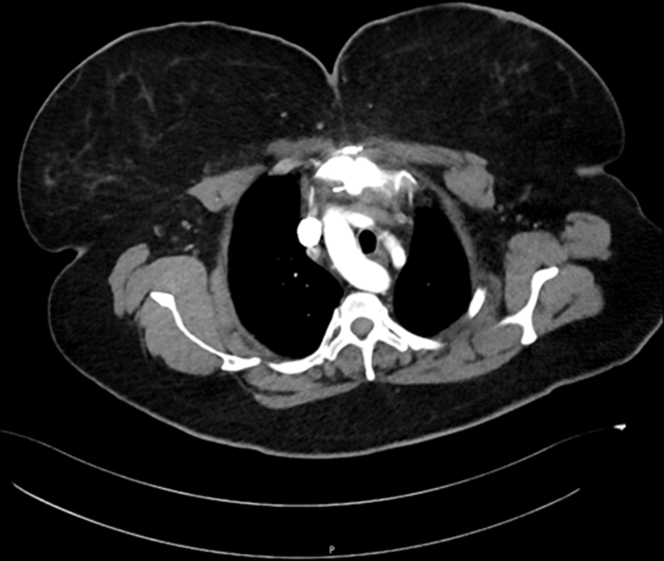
Computed Tomography Scan 6 Months Postoperative Noting Ligation of the Distal Aortic Arch Without Patency
